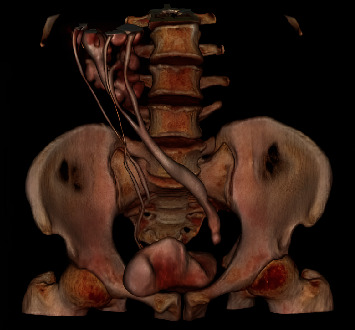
Three-dimensional CT reconstruction showed a crossed-fused ectopia, where the left kidney is fused to the lower renal pole of the right kidney.
